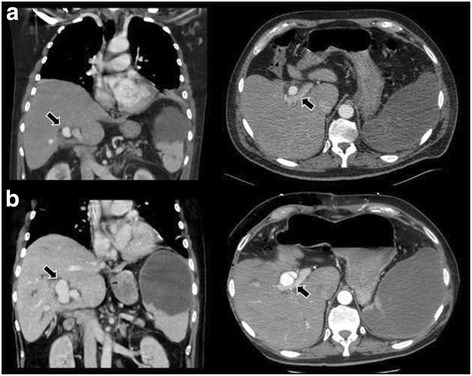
Abdominal CT taken on day 3 (a) shows MA of the proximal right hepatic artery of 1.4 cm × 1.1 cm (*arrow*). Moreover the diagnostic imaging discloses a nonenhancing hypodense area of the spleen consistent with SI. Abdominal enhanced CT taken on day 20 (b) reveals an enlargement of the previously detected MA of the proximal right hepatic artery (*arrow*) and the development of a new large MA of the VIII segment (*arrow*). The radiological findings confirm SI
